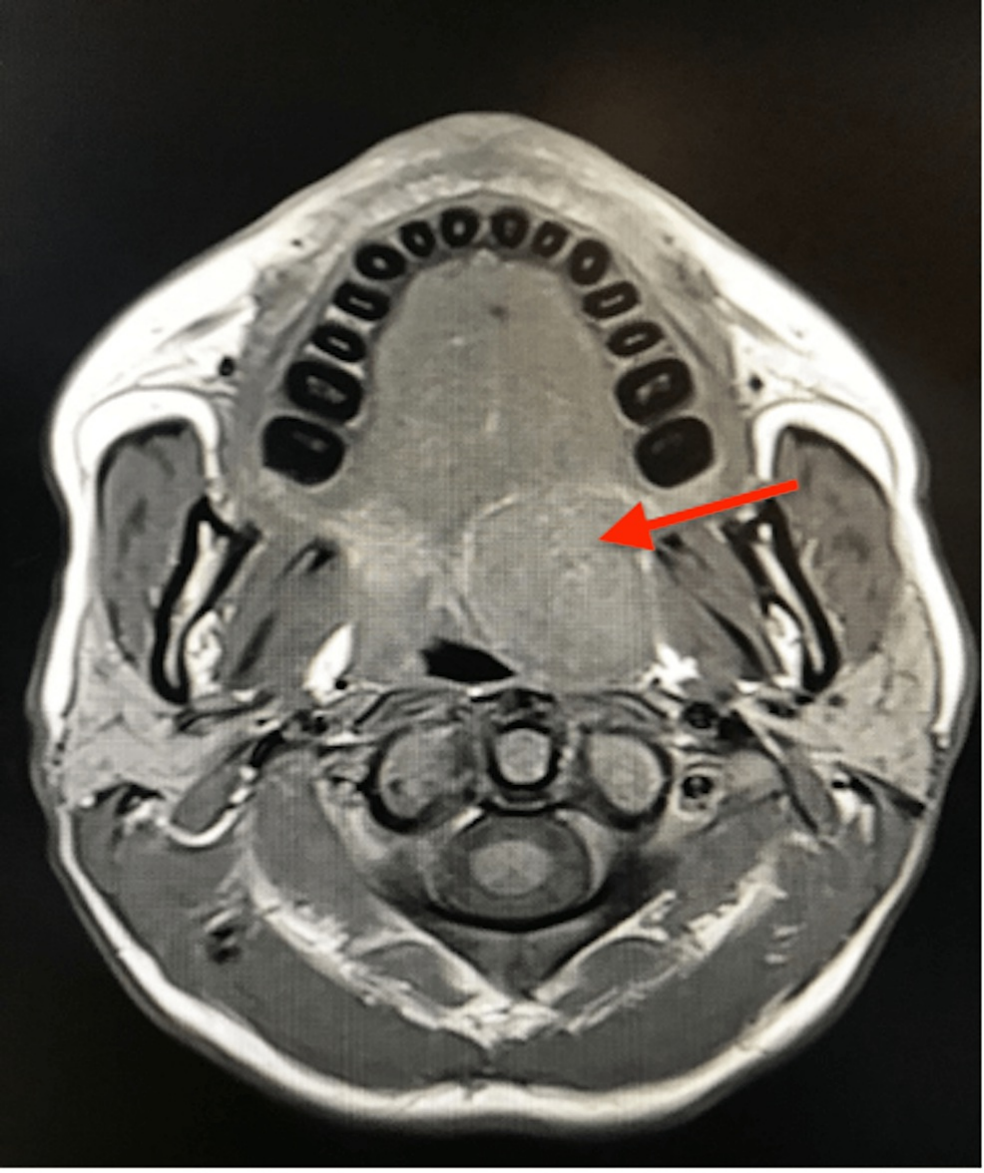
Magnetic resonance imaging in the T2 axial section showing a voluminous expansive process evaluated at 28 × 26 × 27 mm located at the junction of the soft palate and the left palatine tonsil presenting a low signal close to the signal of the tonsil but slightly more heterogeneous (red arrow).
